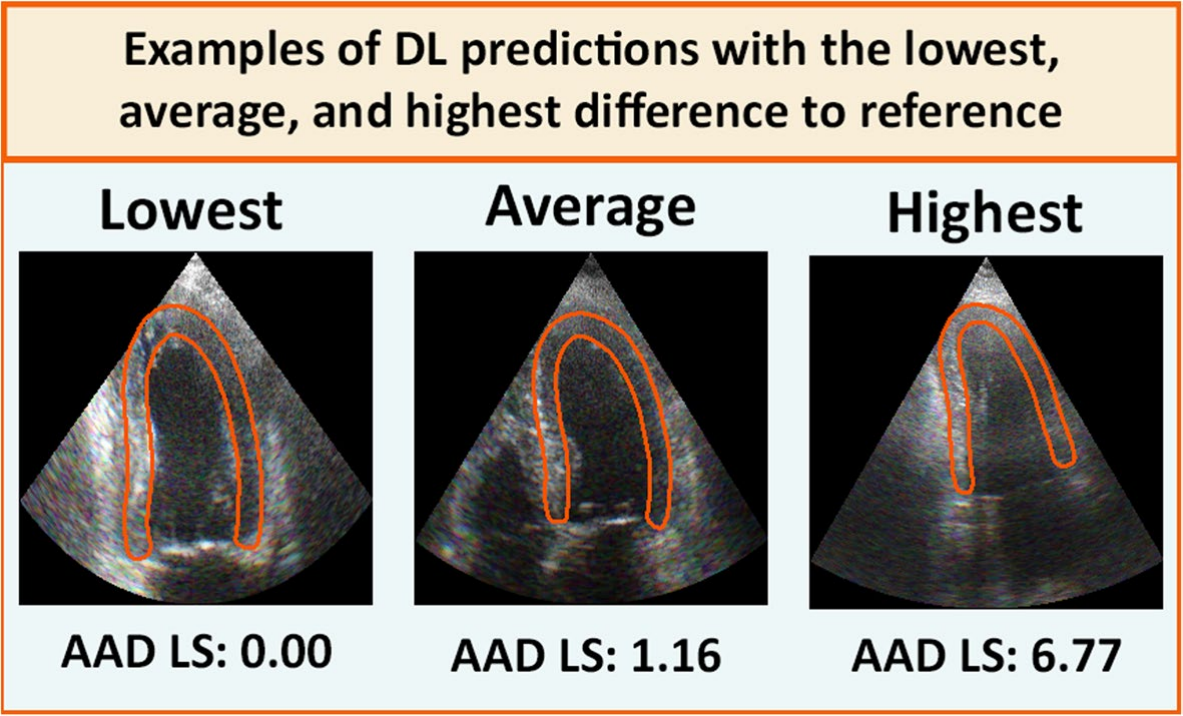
Examples of DL predicted ROI overlaid on corresponding echocardiographic image AAD = Average absolute difference, LS = Single projection longitudinal strain
